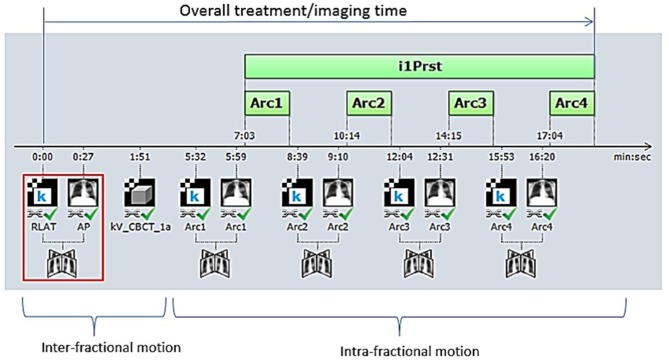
Our image-guided work flow during the treatment of stereotactic body radiation therapy for prostate cancer. Initially, patient positioning is accomplished by orthogonal image pair or stereoscopic X-ray imaging with ExacTrac® and cone-beam computed tomography (CBCT) before treatment beam-on. Repeat orthogonal image pair or stereoscopic X-ray imaging is obtained prior to each beam delivery (each of three remaining half-arcs) to account for intra-fractional motion. Each half-arc is delivered over ~2–3 min.
